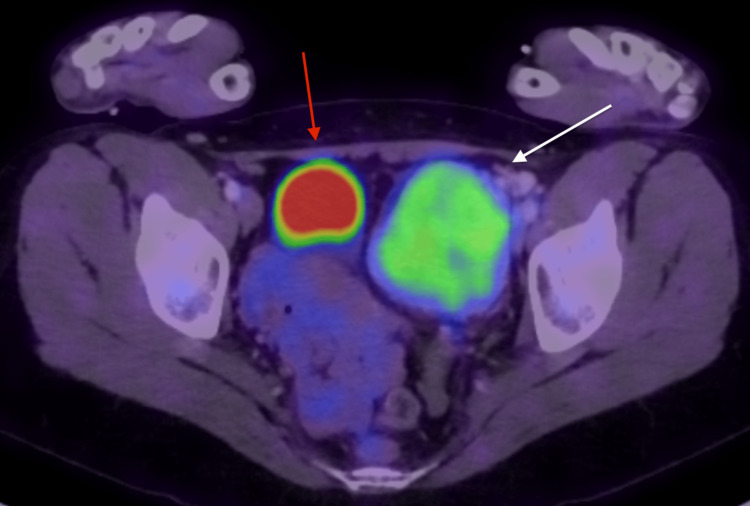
PET/CT showing FDG-avid left pelvic wall mass (white arrow) and bladder (red arrow) PET/CT: Positron emission tomography/computed tomography; FDG: Fludeoxyglucose
